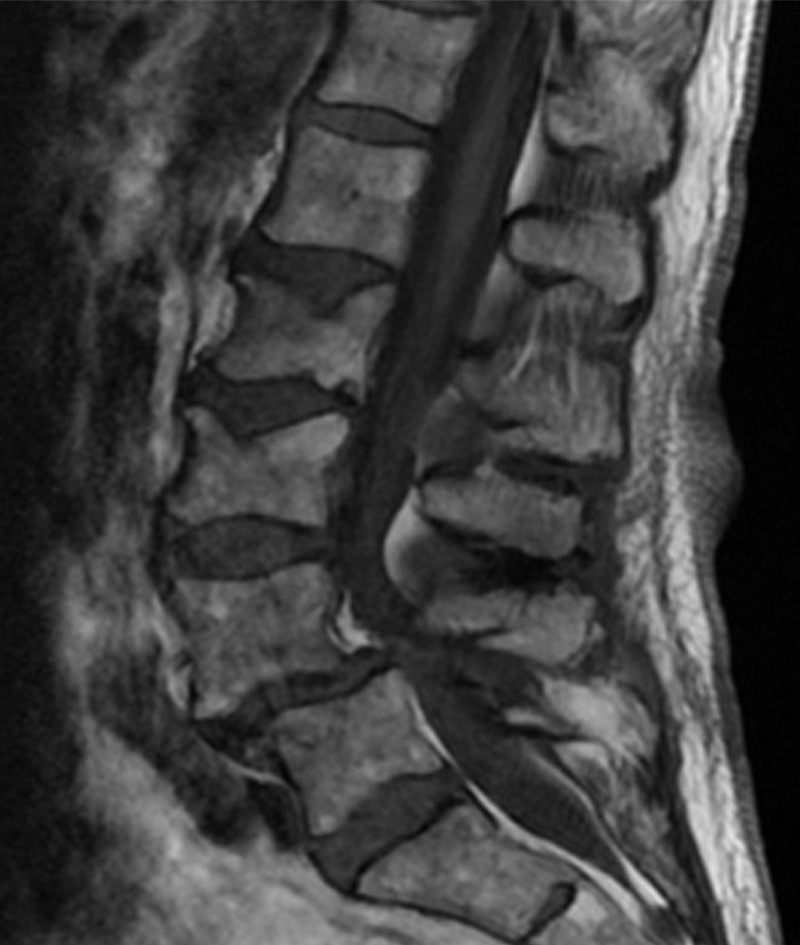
MRI of a Poor Candidate This MRI shows features of a poor candidate for indirect decompression. Note the high-grade central canal stenosis.
